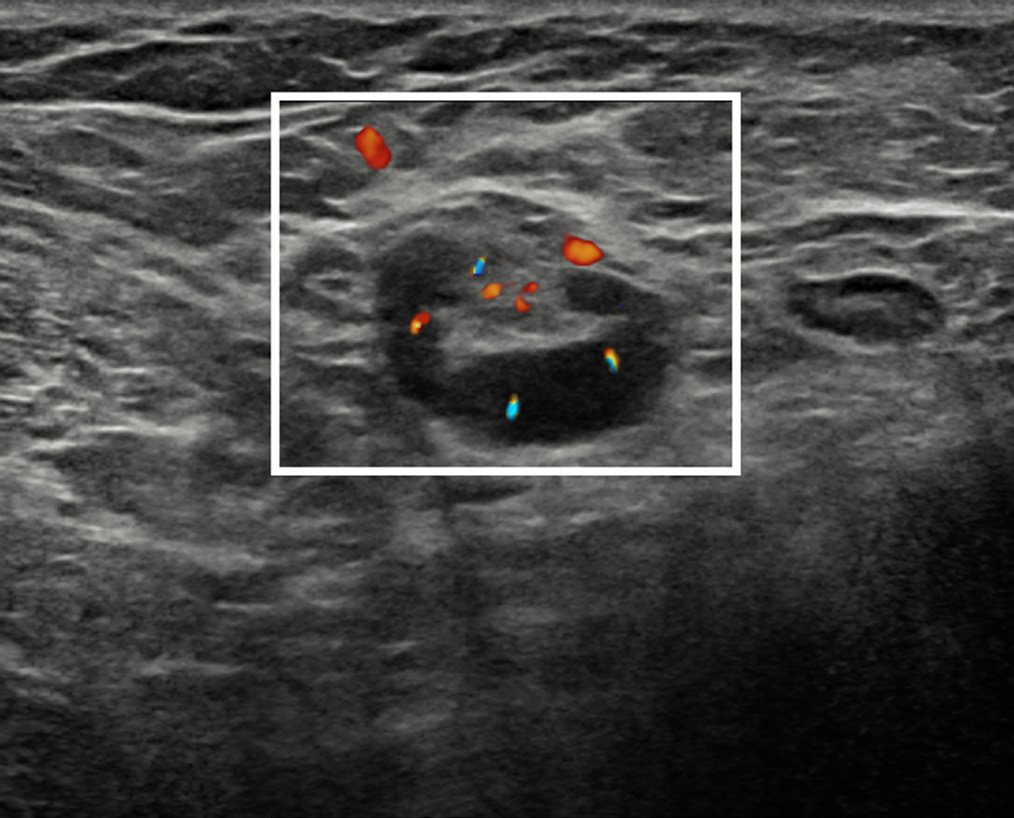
Ultrasound scan with Doppler signal overlapped on B-mode images of the right armpit showing an enlarged lymph node with increased intra- and extranodal vascularization and congested hilum (white rectangle), suggestive for active inflammation.
